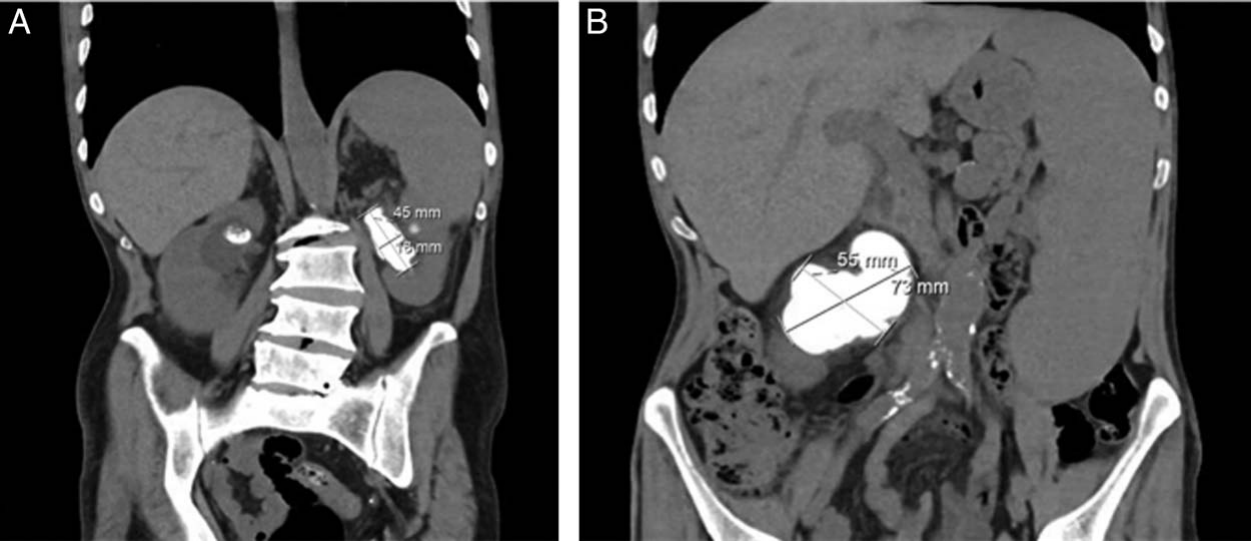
Coronal view computed tomography scan showing two large staghorn calculi, with compression of the inferior vena cava on the right.
